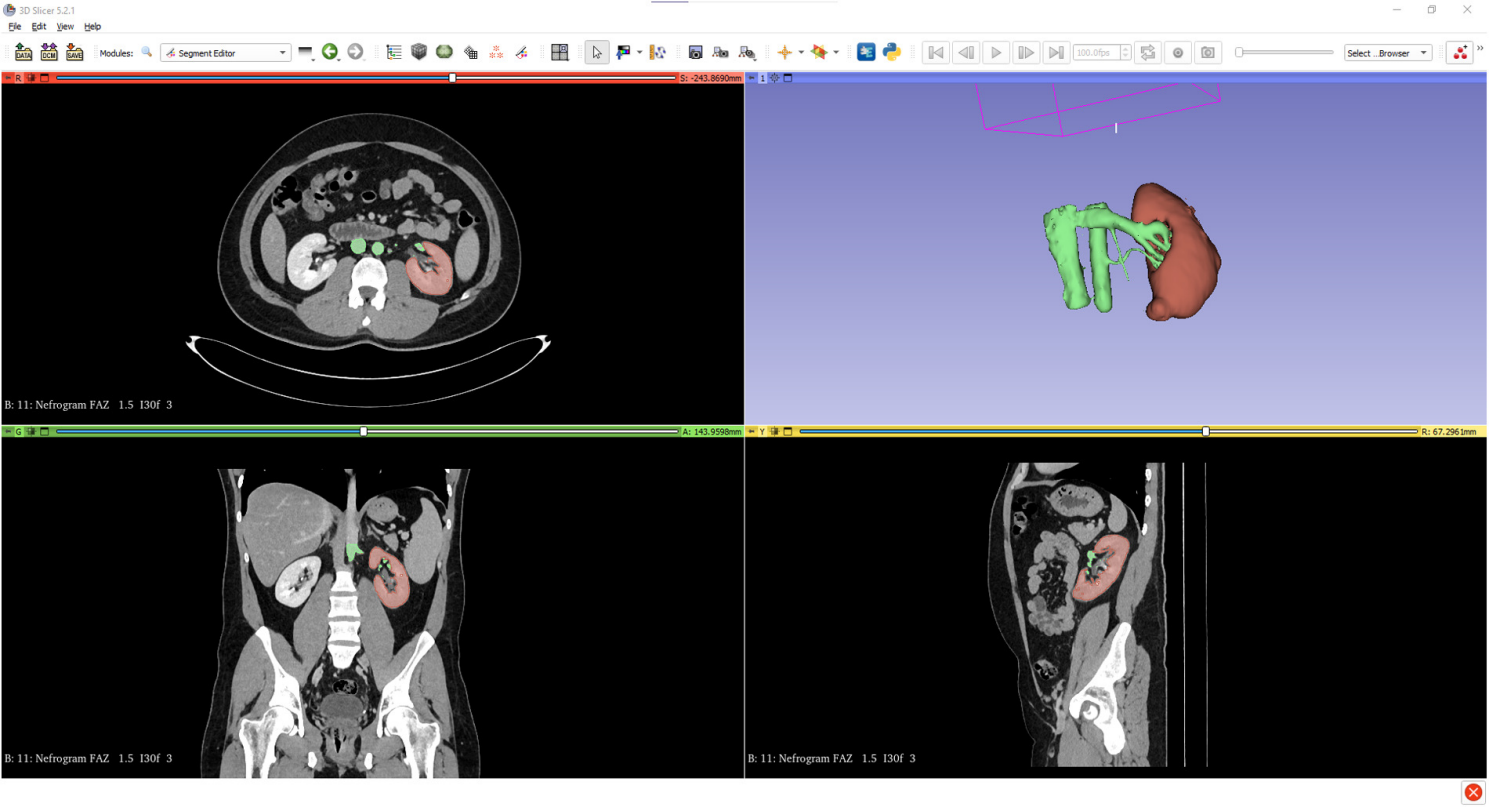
Segmentation images of the kidney tumor generated from CT scans using 3D Slicer software.
